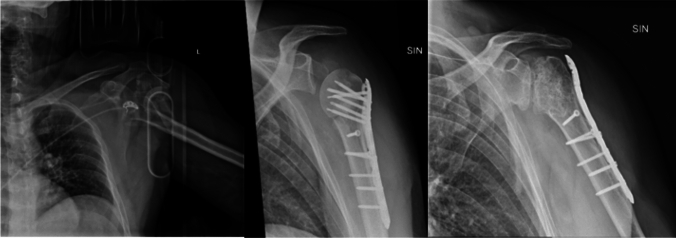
X-rays of a comminuted displaced 4-part proximal humerus fracture in a 47-year-old man following a motor bike accident that led to AVN at 1-year post-op and was treated with proximal screws removal
